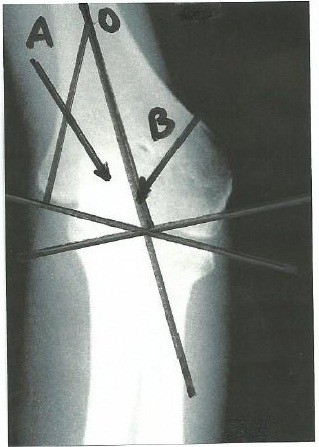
Follow-up radiographs 5 years after fracture of a cubitus varus elbow. The TCI is greater than 1.
